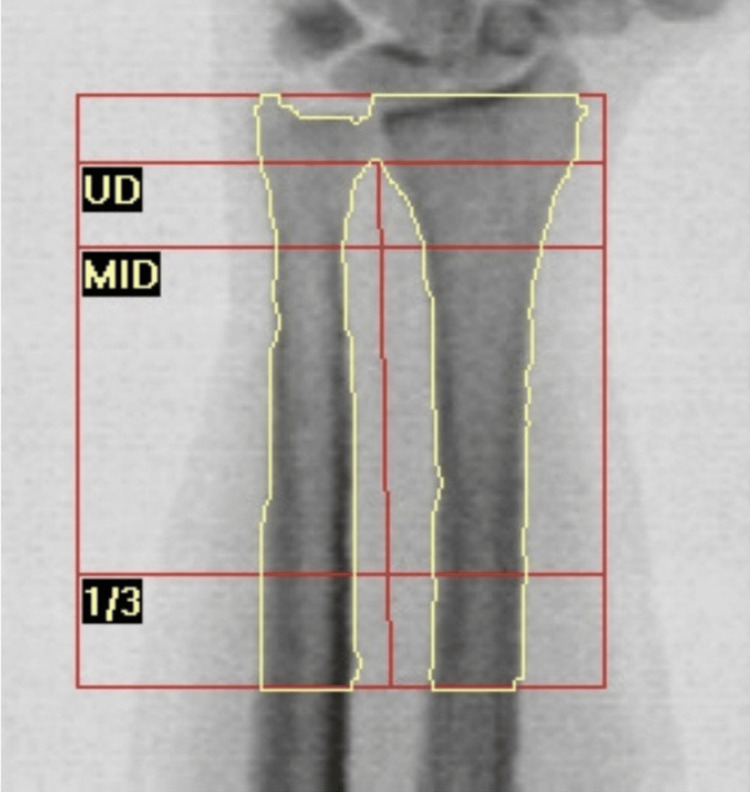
Dual-energy X-ray absorptiometry scan demonstrated severe osteoporosis at the distal forearm with a T-score of -3.5. Primary hyperparathyroidism characteristically shows predominant involvement of the distal forearm, owing to the high proportion of cortical bone at this site.
